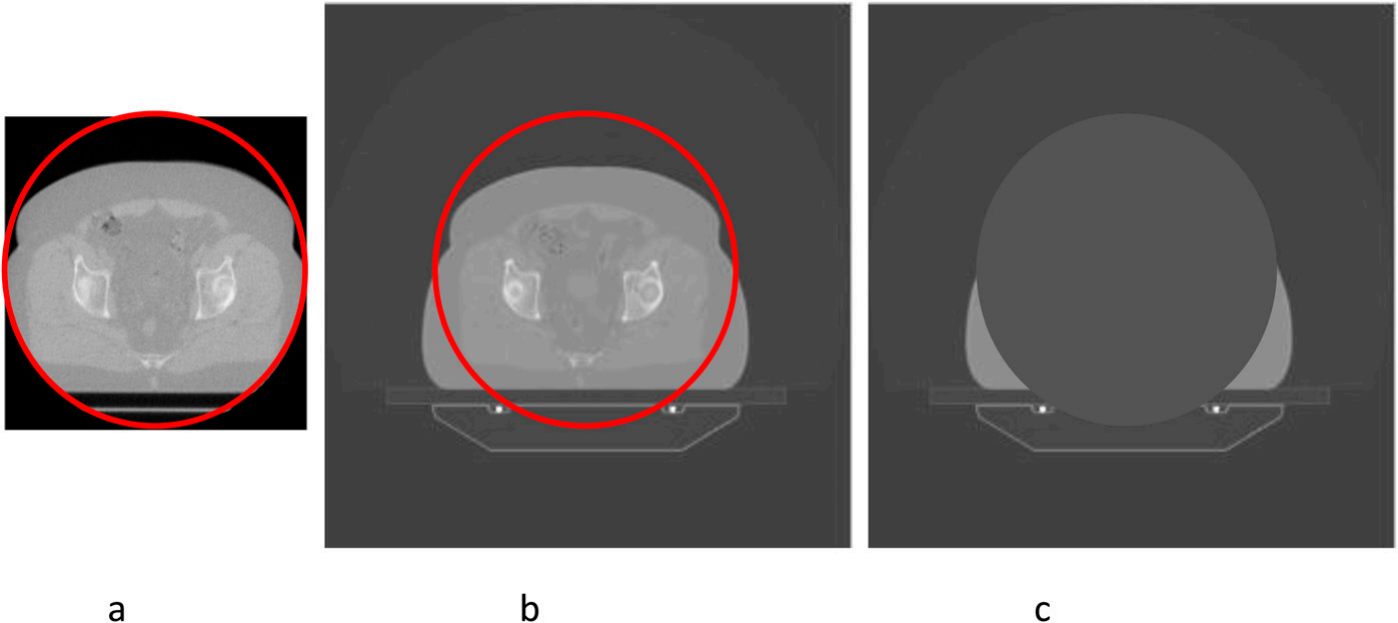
Combination of kilovoltage (kV) CT and megavoltage (MV) CT images. (a) The MV CT image, with the scan circle shown in red. (b) This circle superimposed on the aligned kV CT image. (c) The masked image, in which the area within the scan circle has been assigned zero density. The radiological path length to each point is the sum of the radiological path lengths from the first and third images. For colour see online.
